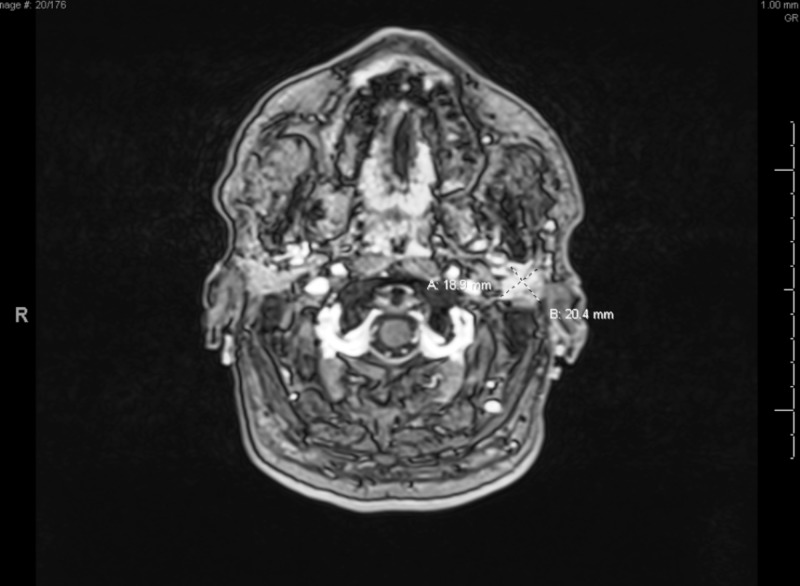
CT image of the patient's symptomatic adenocarcinoma tumor. This left-sided 2x2cm lesion caused facial droop, sensory deficits, and hearing loss.
